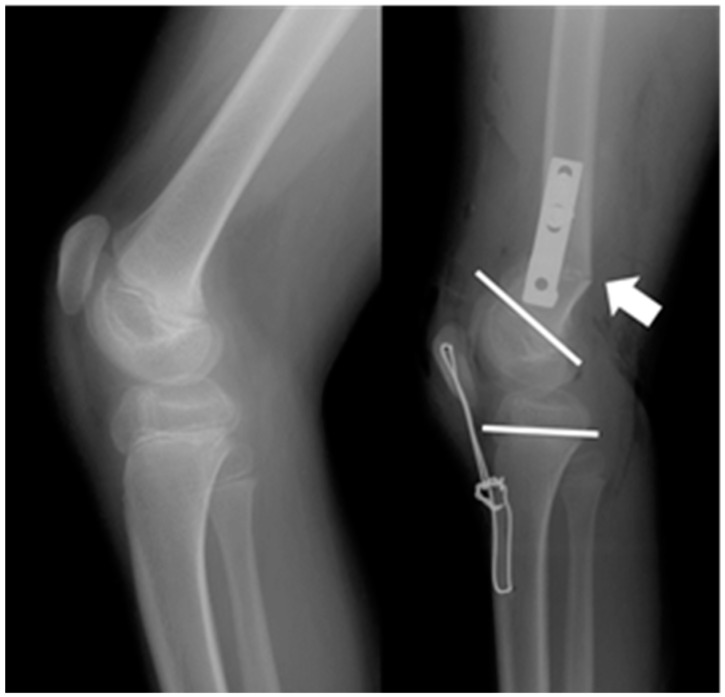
Preoperative and postoperative radiographs of a patient whose preoperative knee flexion contracture measured 43°. After resection of a wedge-shaped bone via a distal femoral extension osteotomy, significant angular deformity developed at the distal femur. Difficulty in securing fixation with a plate may be encountered and the weight-bearing surface of the distal femur may shift to the posterior condyles during the mid-stance phase of the gait.
